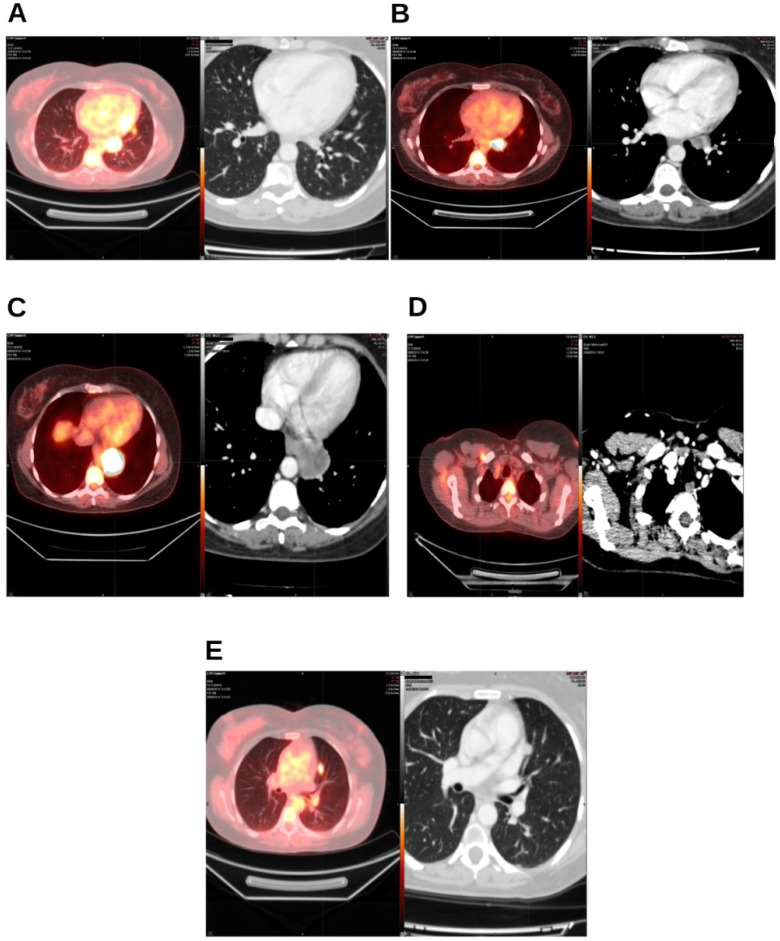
Diagnostic tests before the start of radiotherapy treatment. On the left, the PET-TC from August 2019; on the right, the CT from August 2019. (A) Lingular segment; (B) left hilar lymph node; (C) left paraesophageal nodule; (D) right retropectoral lymph node; (E) nodule in left parascissural segment.
